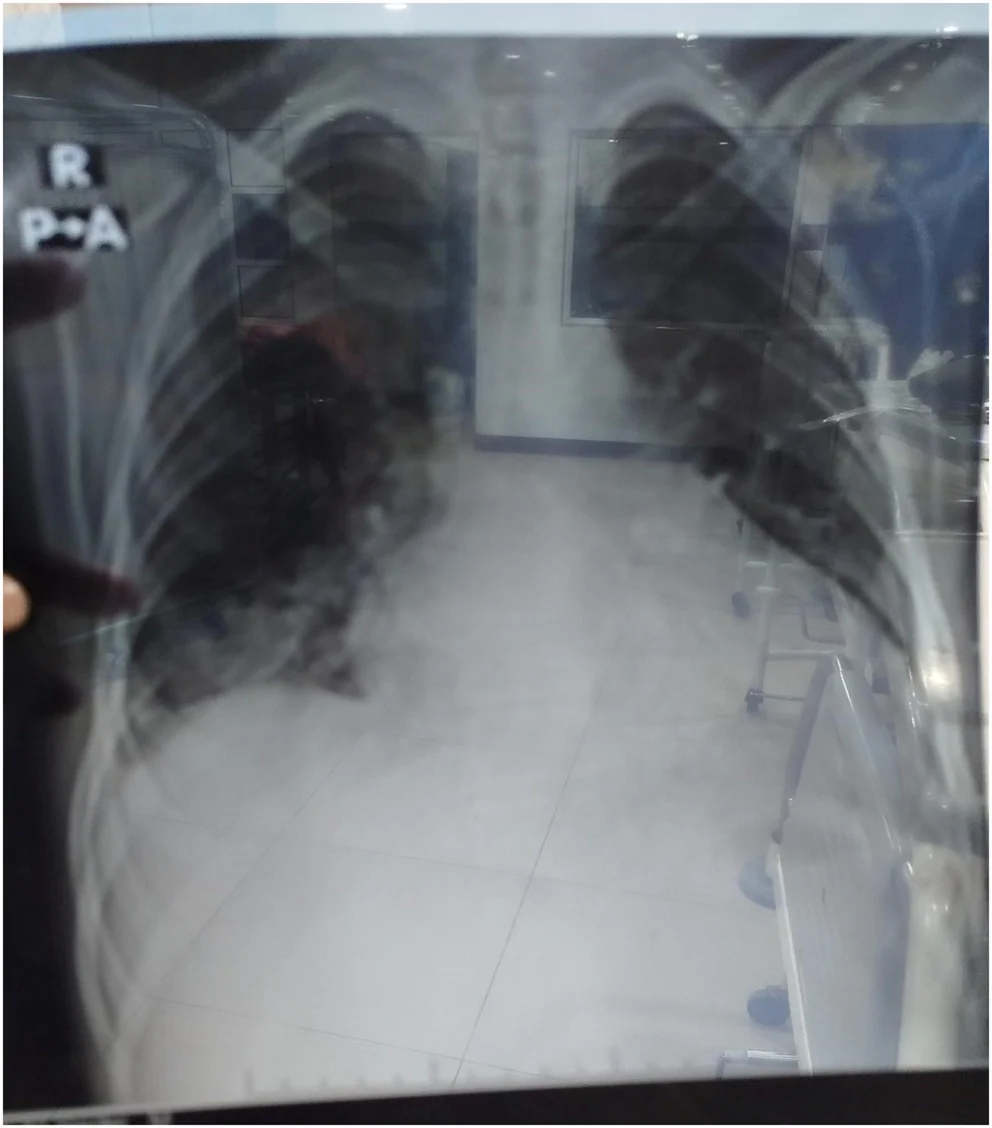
Posteroanterior chest radiograph demonstrating cardiomegaly with prominence of the central pulmonary arteries and increased pulmonary vascular markings, consistent with chronic pulmonary hypertension.
